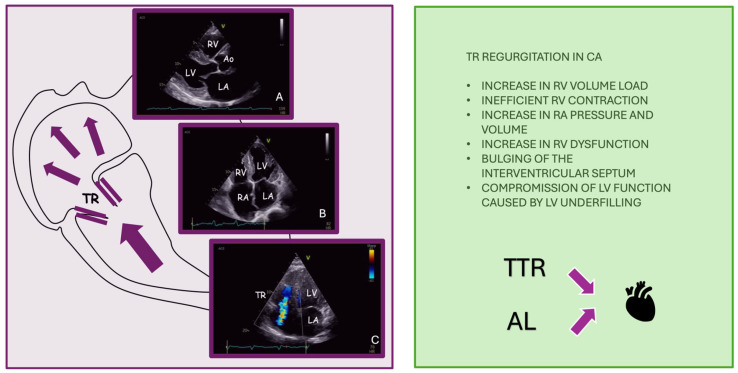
(A) the trans-thoracic echocardiogram (parasternal long axis view) demonstrates concentric myocardial hypertrophy and right ventricle free wall thickening (indirect sign of pulmonary hypertension). (B) the trans-thoracic echocardiogram (apical 4 chamber view) shows the sparkling, granular appearance of the myocardium. (C) the trans-thoracic echocardiogram (apical 4 chamber view) represents moderate tricuspid regurgitation by color Doppler assessing. Abbreviations: LV: left ventricle, RA: right atrium, RV: right ventricle, TR: tricuspid regurgitation, Ao: aortic valve, LA: left atrium, TTR: transthyretin, AL: amyloidosis AL.
